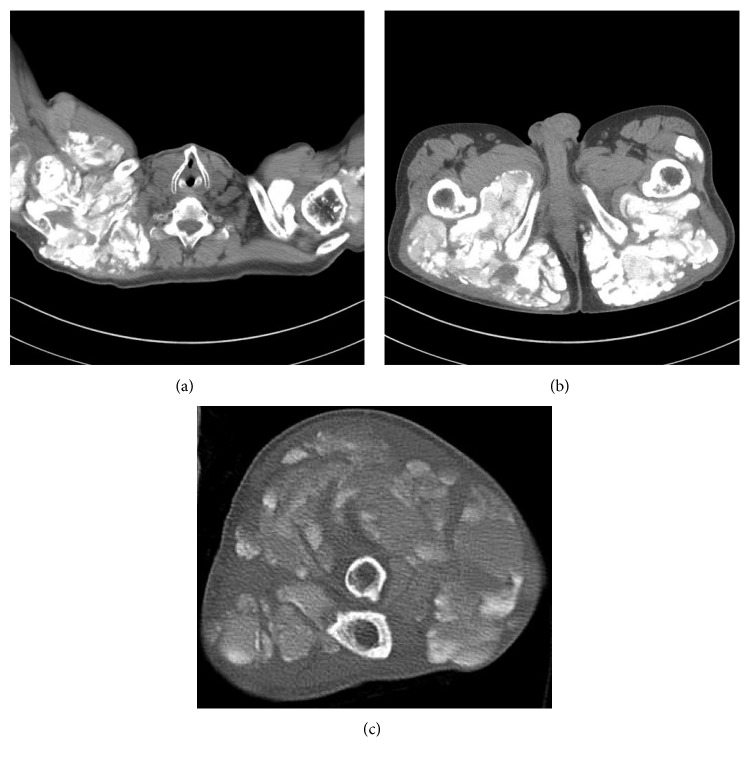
Computed tomography findings at the shoulders (a), hips (b), and left elbow (c).
